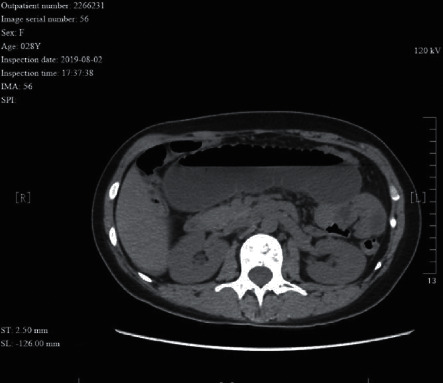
A 28 year-old female patient had received “appendectomy” and suffered from “intestinal obstruction” for several times after the operation. The CT image shows a fish tooth sign. Partial ileum and its mesenterium adhered to the abdominal wall of the incision, and no bowel wall ischemia was identified during surgery.
